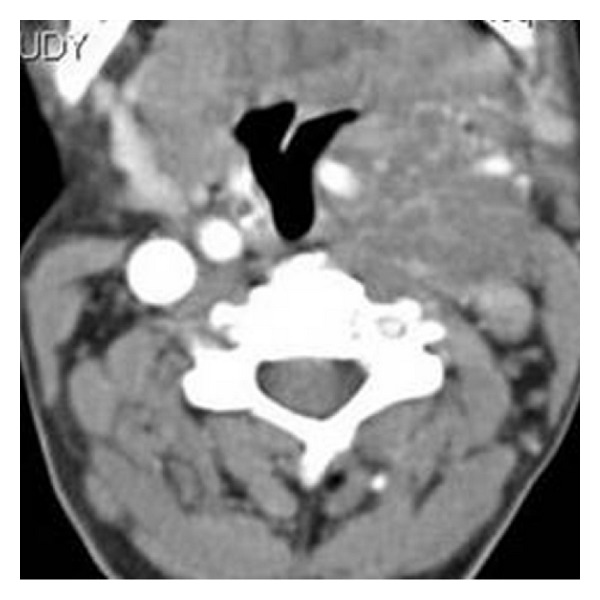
On CT scan, neck with contrast reveals a predominantly isodense mass on the left parapharyngeal space showing mild heterogeneous contrast enhancement. Note the insinuation of the mass between internal and external carotid arteries with resultant splaying of internal and external carotid arteries.
